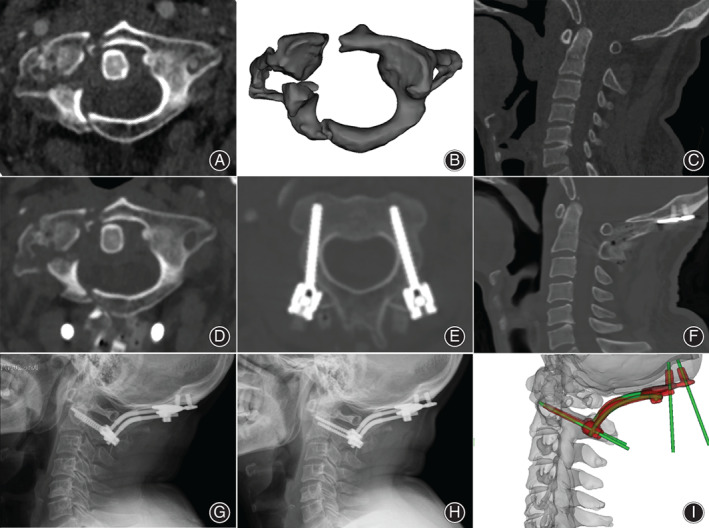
The imaging of patient NO. 10, male, diagnosed with a fracture of the lateral mass of C_1_, type 4. (A) Axial CT of C_2_; (B) 3D imaging of C_2_; (C) Pre‐operation O‐C_2_ angle of 6.71°; (D) postoperative atlas reduction; (E) C_2_ pedicle screw with grade 0; (F) occipital screw with grade 0; (G) OCI angle of 79.56° and O‐C_2_ angle of 14.12° immediately post‐operation; (H) OCI angle of 77.45° and O‐C_2_ angle of 14.79° at the last follow‐up; (I) registration of post‐ and pre‐operation CT, screw placement and occipitocervical angle consistent with the preoperative design.
